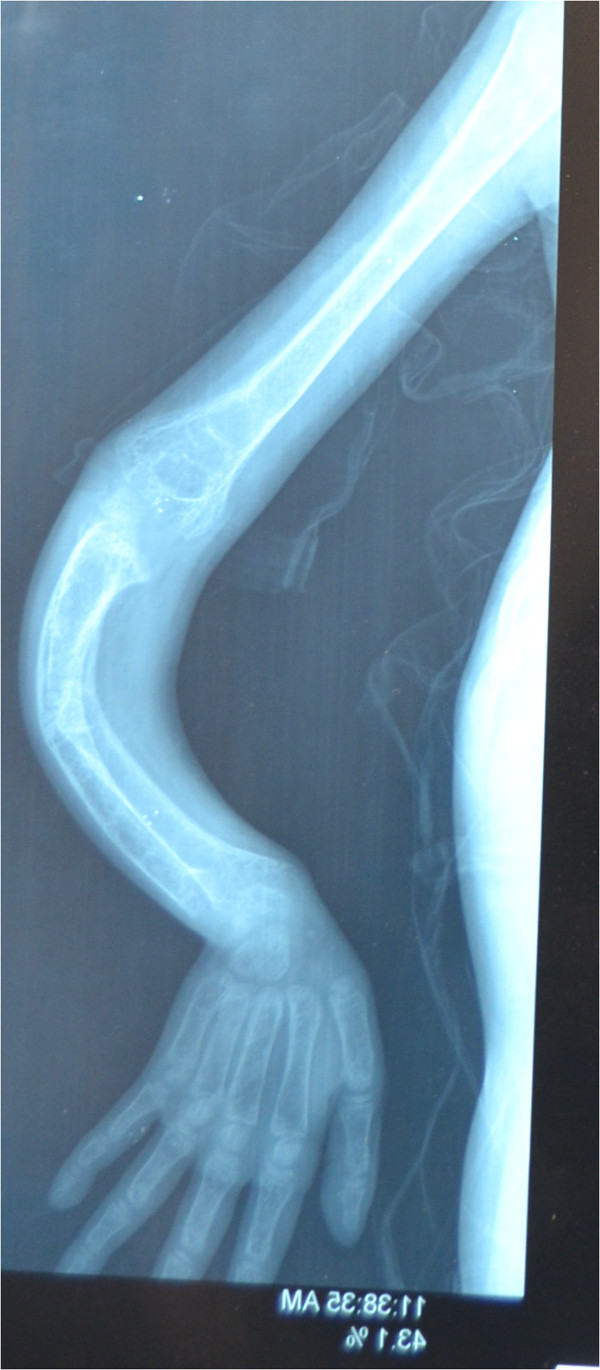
X-ray of the patient’s upper limb showing diffuse osteopenia and bowing of forearm with blurring of the metaphyseal lines. It also shows dense transverse lines in the ulna suggestive of looser’s zones indicative of severe rickets.
